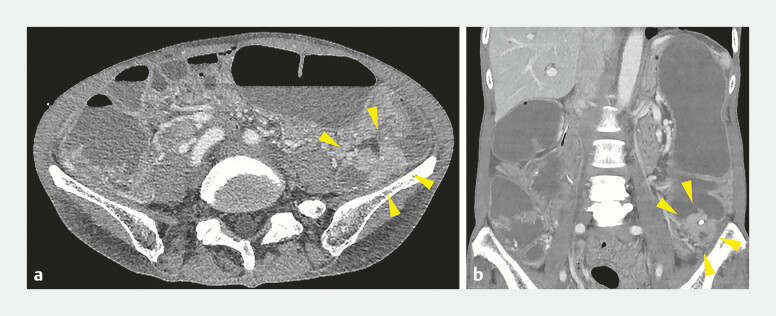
Abdominal contrast-enhanced computed tomography images. Horizontal (
